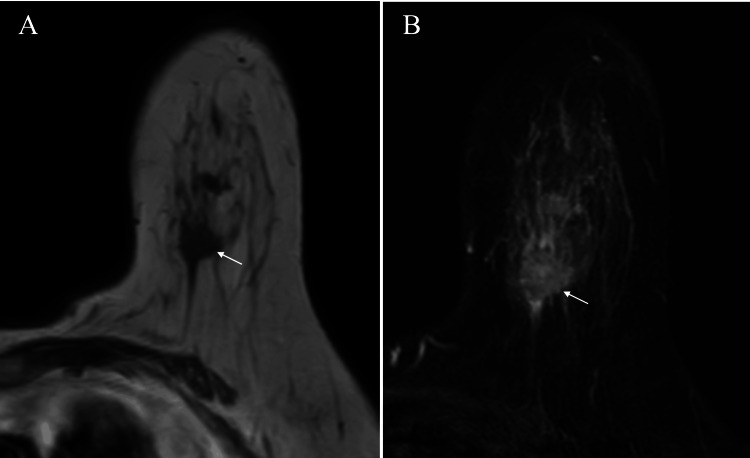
MRI findings MRI of the tumors showed low signals on T1-weighted images (A, arrow) and weakly high signals on fat-suppressed T2-weighted images (B, arrow), highly suggestive of fibrous components within the masses.
